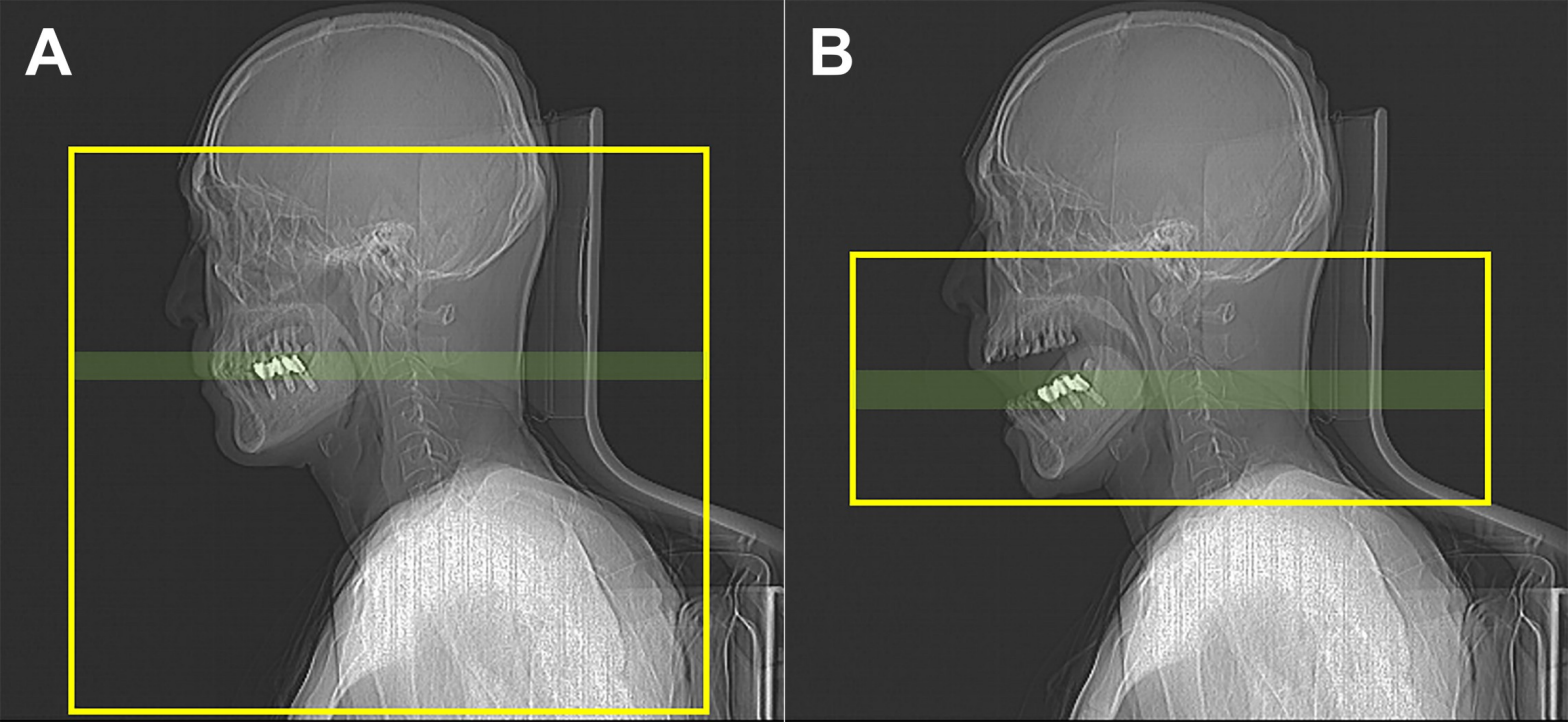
Field-of-view (yellow box) and imaging plane (green box) on lateral scout images of conventional (A) and open mouth (B) computed tomography.
